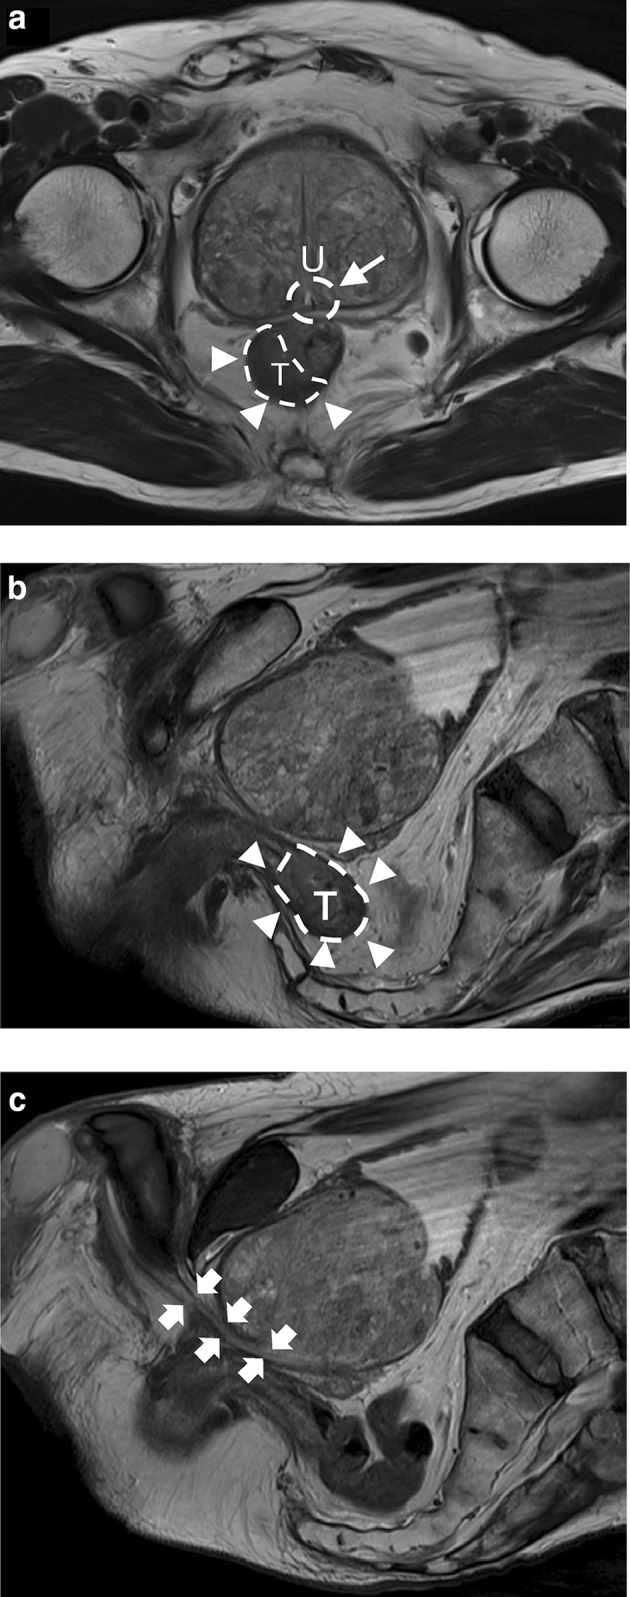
T2-weighted image of MRI. a Axial section. The urethra (*white arrow*) was just above the tumor (*white arrowhead*). *T* tumor, *U* urethra. b Midline sagittal section. The tumor (*white arrowhead*) was located 3 cm above the anal verge. *T* tumor. c Sub midline sagittal section. The urethra (*white arrow*) was deviated by a huge prostate
